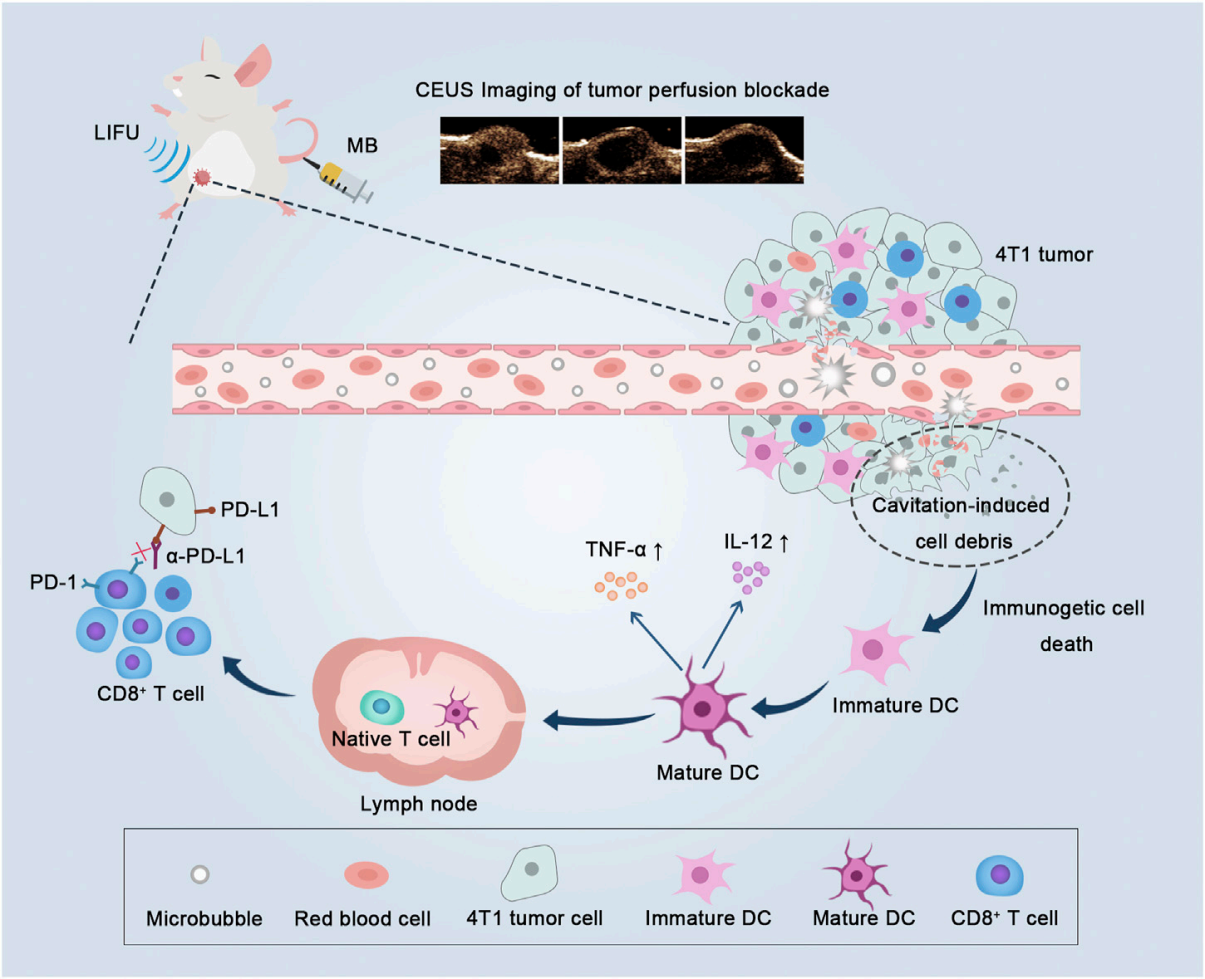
Low-intensity focused ultrasound-targeted microbubble destruction (LIFU-TMD) damages tumor vascular endothelial cells and starves tumor cells by cutting off the blood supply, and simultaneously tumor cell debris induced by the cavitation effect further stimulates dendritic cells (DCs) maturation, and ultimately activating CD8^+^ T cells, thereby enhancing the efficacy of PD-L1 blockade.
